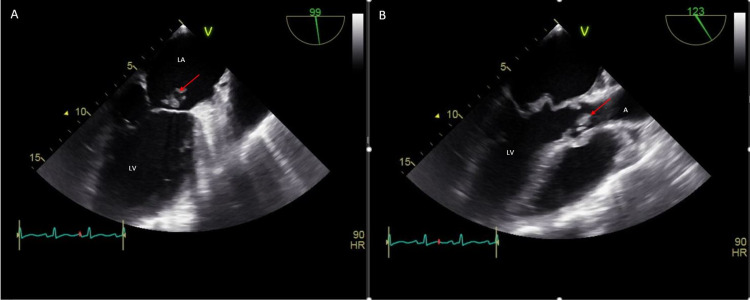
Transesophageal echocardiogram (TEE) images demonstrating a large mitral valve vegetation (A) and a medium-sized aortic valve vegetation (B) LA = left atrium, LV = left ventricle, A = aorta
